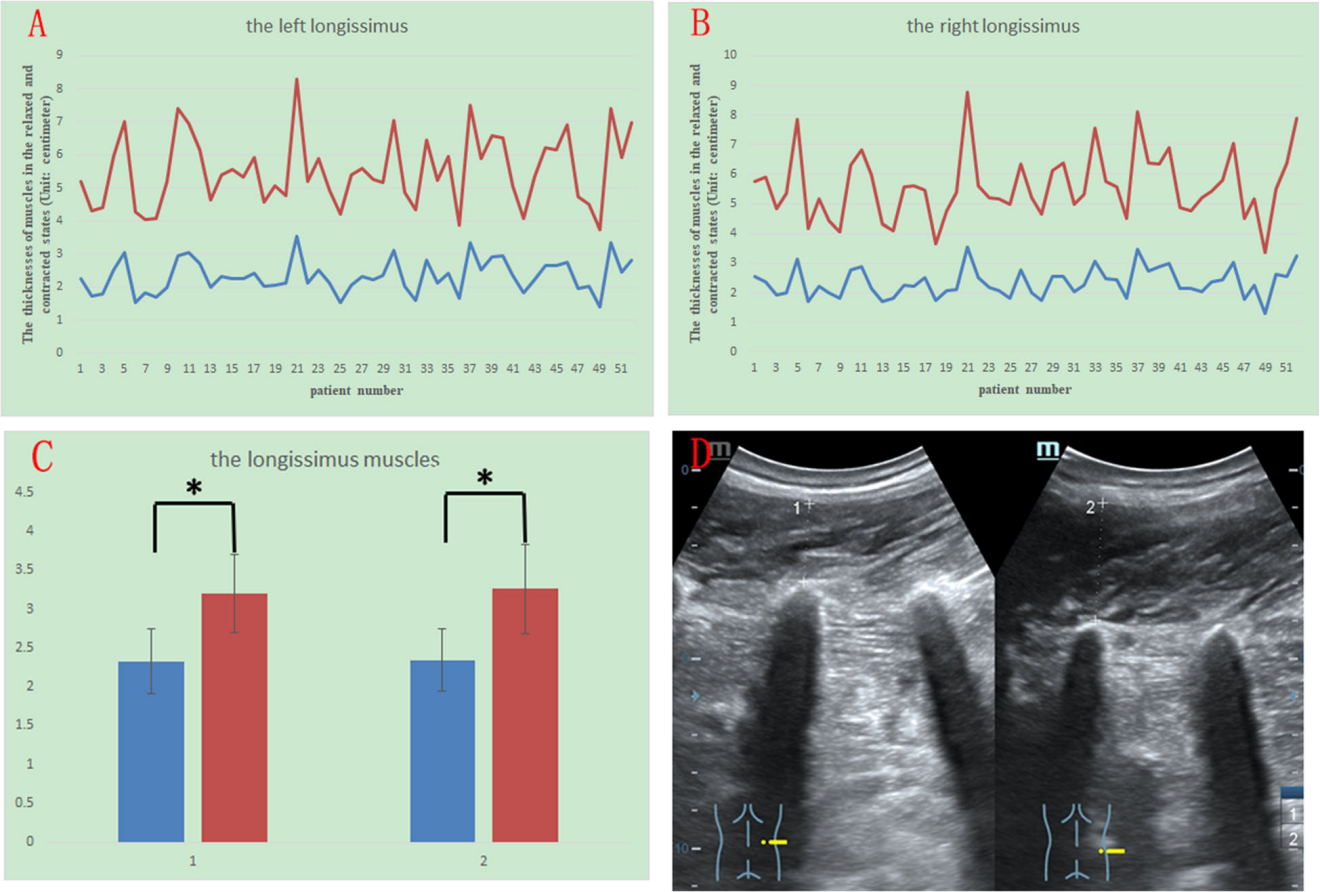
The thicknesses of the left (Fig. 4 A) and right (Fig. 4B) longissimus muscles in the resting (blue line) and contracted conditions (red line). Comparison of the thicknesses in the resting (blue column) and contracted conditions (red column). Group 1 is right, and group 2 is left (Fig. 4 C). The sonogram of the longissimus: left shows the relaxed state, and right shows the contracted state (Fig. 4D)
